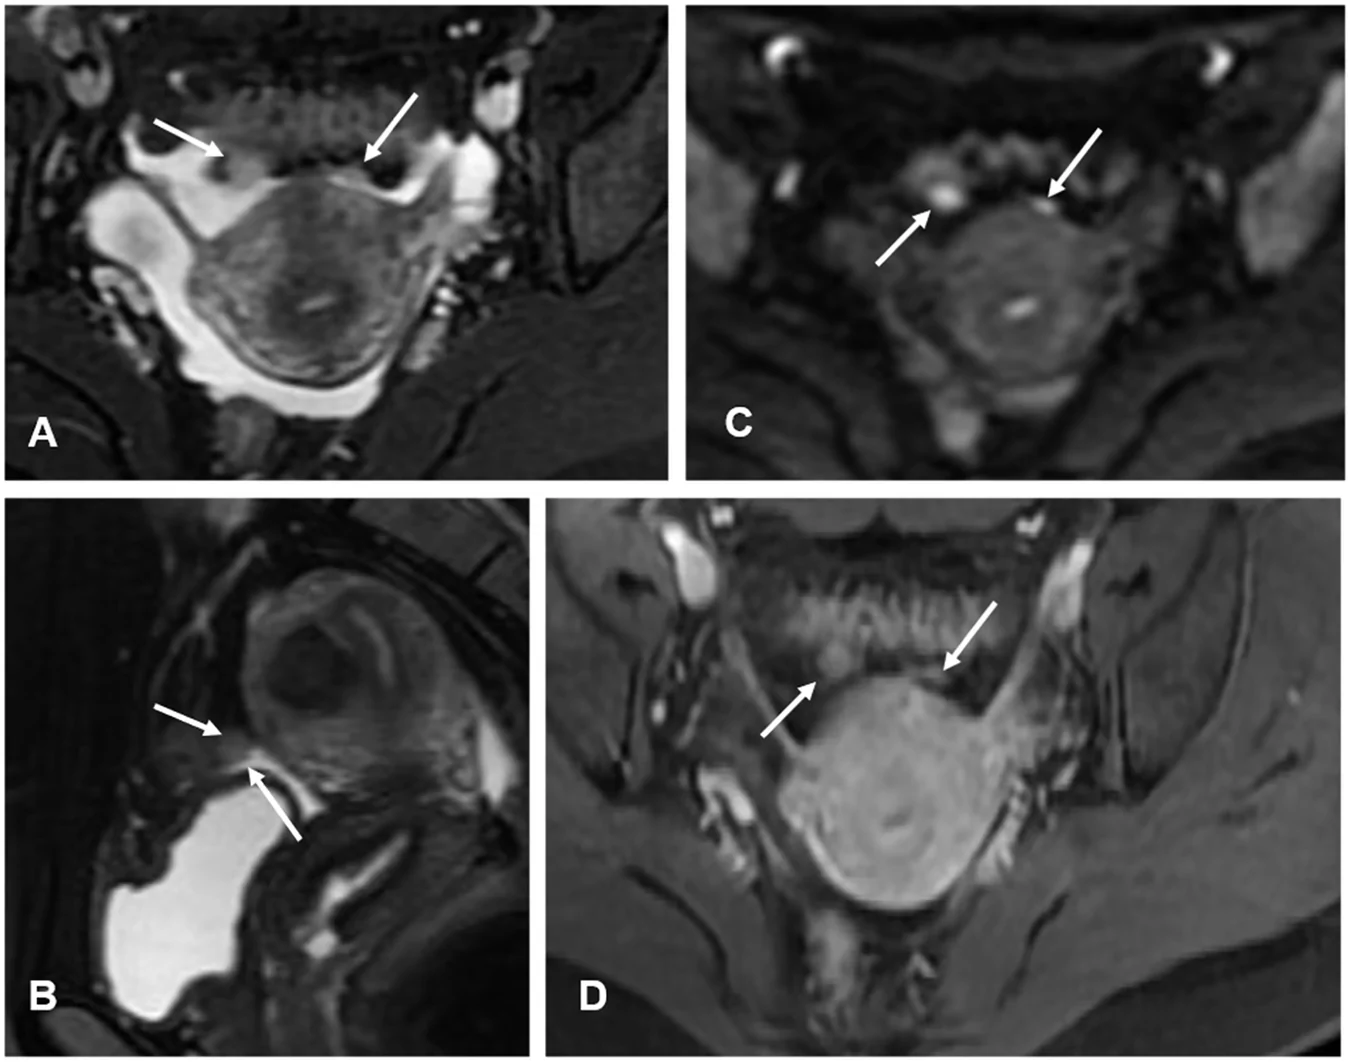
A–D LG-ESS in a 34-year-old woman. Axial (A) and sagittal (B) T2W images showing hyperintense nodules on the serosal surfaces of the bowel and the anterior uterine wall (arrows). Axial DWI image (C) demonstrating markedly hyperintense lesions. Axial T1-weighted contrast-enhanced image (D) showing significant enhancement of the lesions
